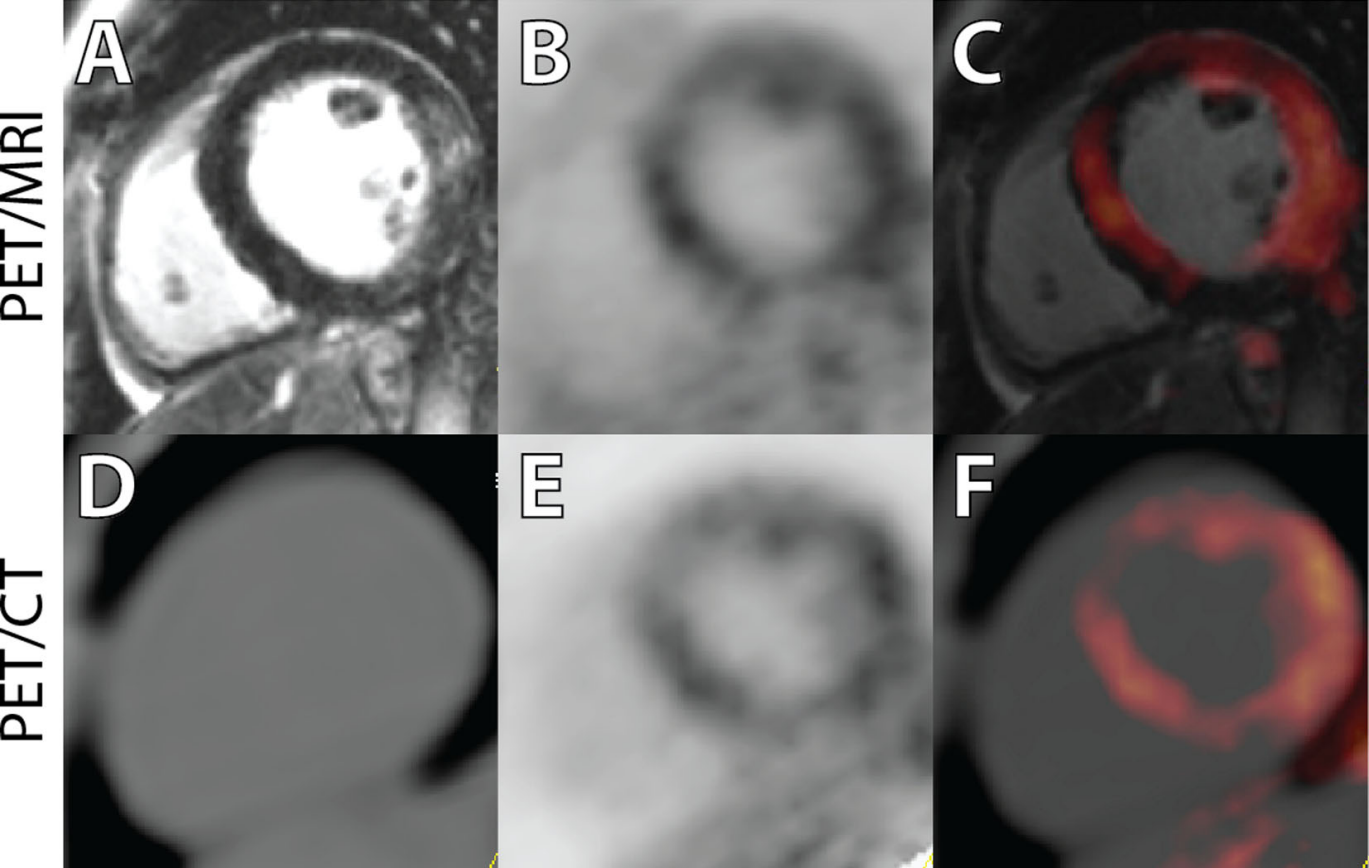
Images presented from Patient 1 with PET/MR (A–C) and PET/CT (D–F). In this patient, note the enhanced signal seen on the delayed enhancement MR images in the lateral wall (A), and the enhanced ^18^F-FDG uptake in the septal, anterior, and lateral regions on both the PET/CT and PET/MR images (C, F). Although this extensive uptake of ^18^F-FDG could be interpreted as poor suppression, an increase in ^18^F-FDG in the hilum and great vessels (not shown in these images) is supportive of this interpretation. This patient had an ejection fraction of 49% with mild global hypokinesis. There were no regional wall motion abnormalities
